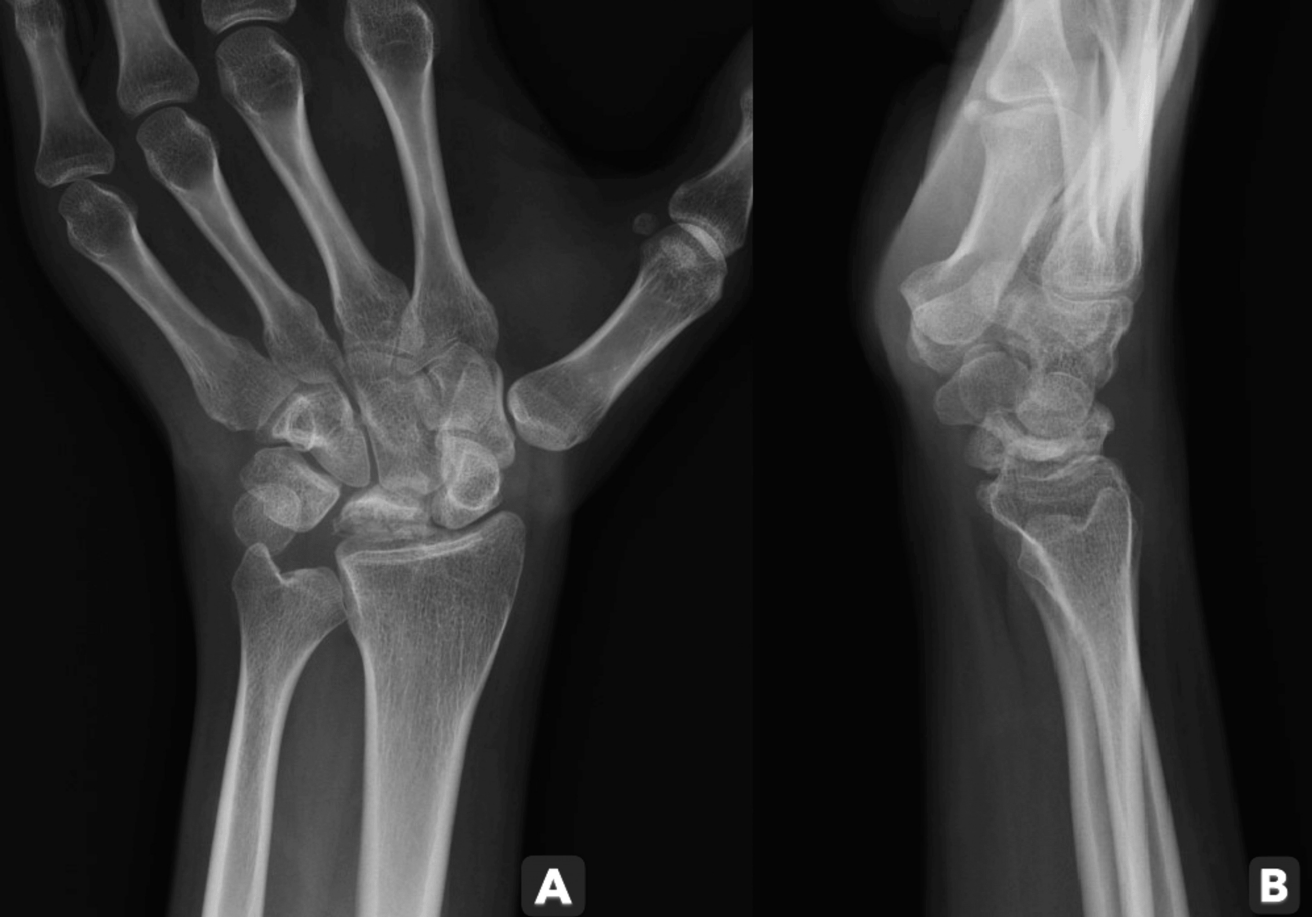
Left wrist radiographs at three-year follow-up with progression of Kienböck's disease. (A) Anteroposterior view. (B) Lateral view.
